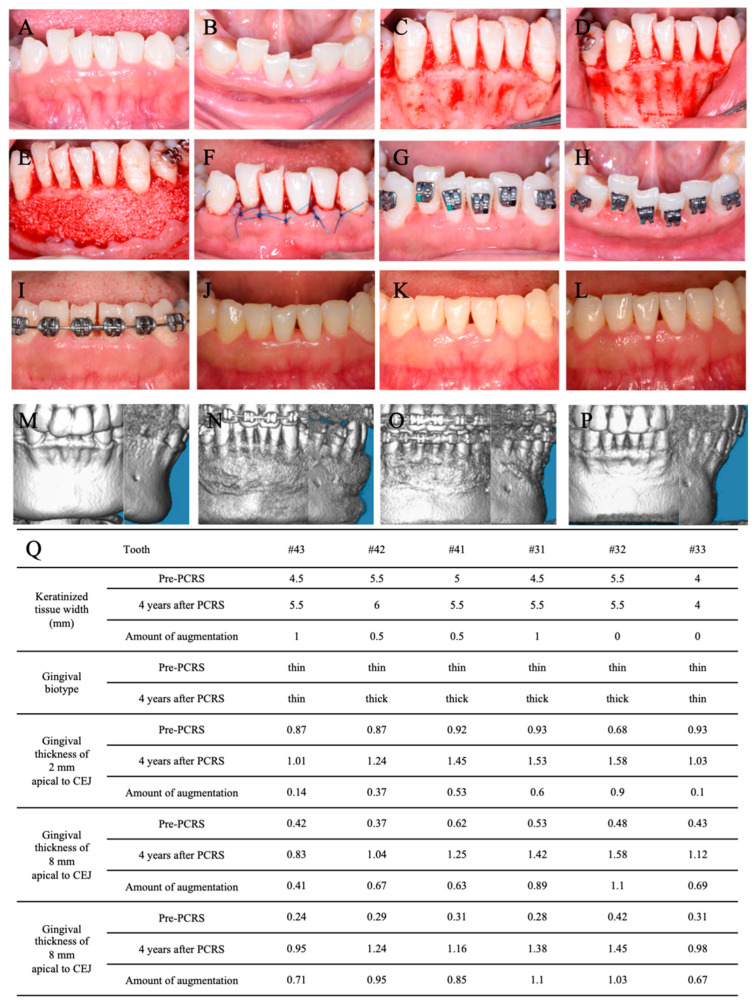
Clinical photographs of PCRS and contrast of pre-PCRS and post-PCRS in the mandibular anterior region. (A) Anterior view before PCRS. (B) Occlusal view before PCRS. (C) The gingival flap was elevated after papillary preservation incisions. (D) Piezoelectric corticotomy. (E) The bone graft was placed on the bone surface. (F) Sutures. Sutures were removed at 2 weeks after PCRS from the anterior view (G) and occlusal view (H). Six months (I), 2 years (J), 3 years (K), and 4 years (L) after PCRS. Three-dimensional reconstruction by CBCT before PCRS (M), immediately (N), 6 months (O), and 4 years (P) after PCRS. (Q) The clinical measurement and contrast of keratinized gingiva width and gingival biotype with gingival thickness before PCRS and 4 years after PCRS.
